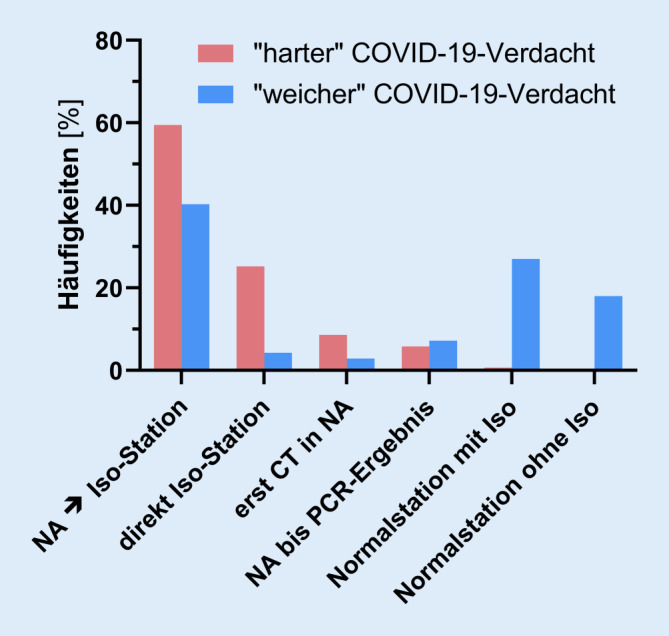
Bei Patienten mit hohem klinischem Verdacht (mutmaßliche COVID-19-Fälle) erfolgt in 59,7 % der teilnehmenden Notaufnahmen (*n* = 83) die initiale Aufnahme in den Räumlichkeiten der Notaufnahme. Anschließend erfolgt eine rasche Verlegung auf eine Isolationsstation. In 25,2 % der Fälle (*n* = 35) werden die Patienten unmittelbar auf die Isolationsstation weitergeleitet und dort aufgenommen. In 8,6 % der Notaufnahmen (*n* = 12) wird zum Zwecke der Allokation noch in der Notaufnahme eine thorakale CT-Bildgebung durchgeführt, bevor über das weitere Prozedere entschieden wird. In 5,8 % der Kliniken (*n* = 8) verbleiben die Patienten zunächst bis zum Ergebnis eines Abstrichs und zum Ausschluss relevanter Begleiterkrankungen in der Notaufnahme. In allen so praktizierenden Notaufnahmen wird ein Verbleib dieser Patienten in der Notaufnahme als sinnvoll erachtet. Eine primäre Aufnahme auf die Normalstation mit dortiger Isolation erfolgt lediglich in 0,7 % (*n* = 1) der teilnehmenden Krankenhäuser.
